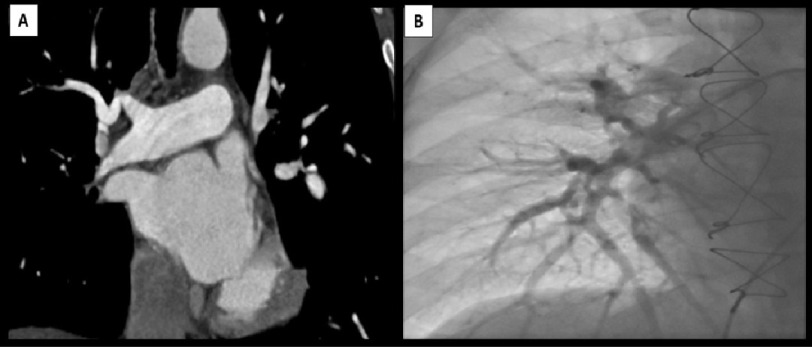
(A) Follow up computed tomography pulmonary angiography
